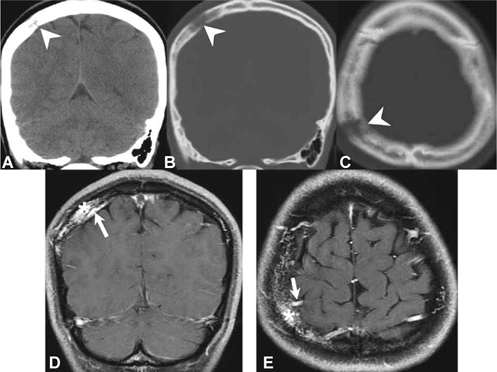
(A-C) A parietal osteolytic lesion on brain CT. (D, E) The enhanced lesion (asterisk) with a feeding artery (arrow) revealed by gadolinium-enhanced T1-weighted imaging.
